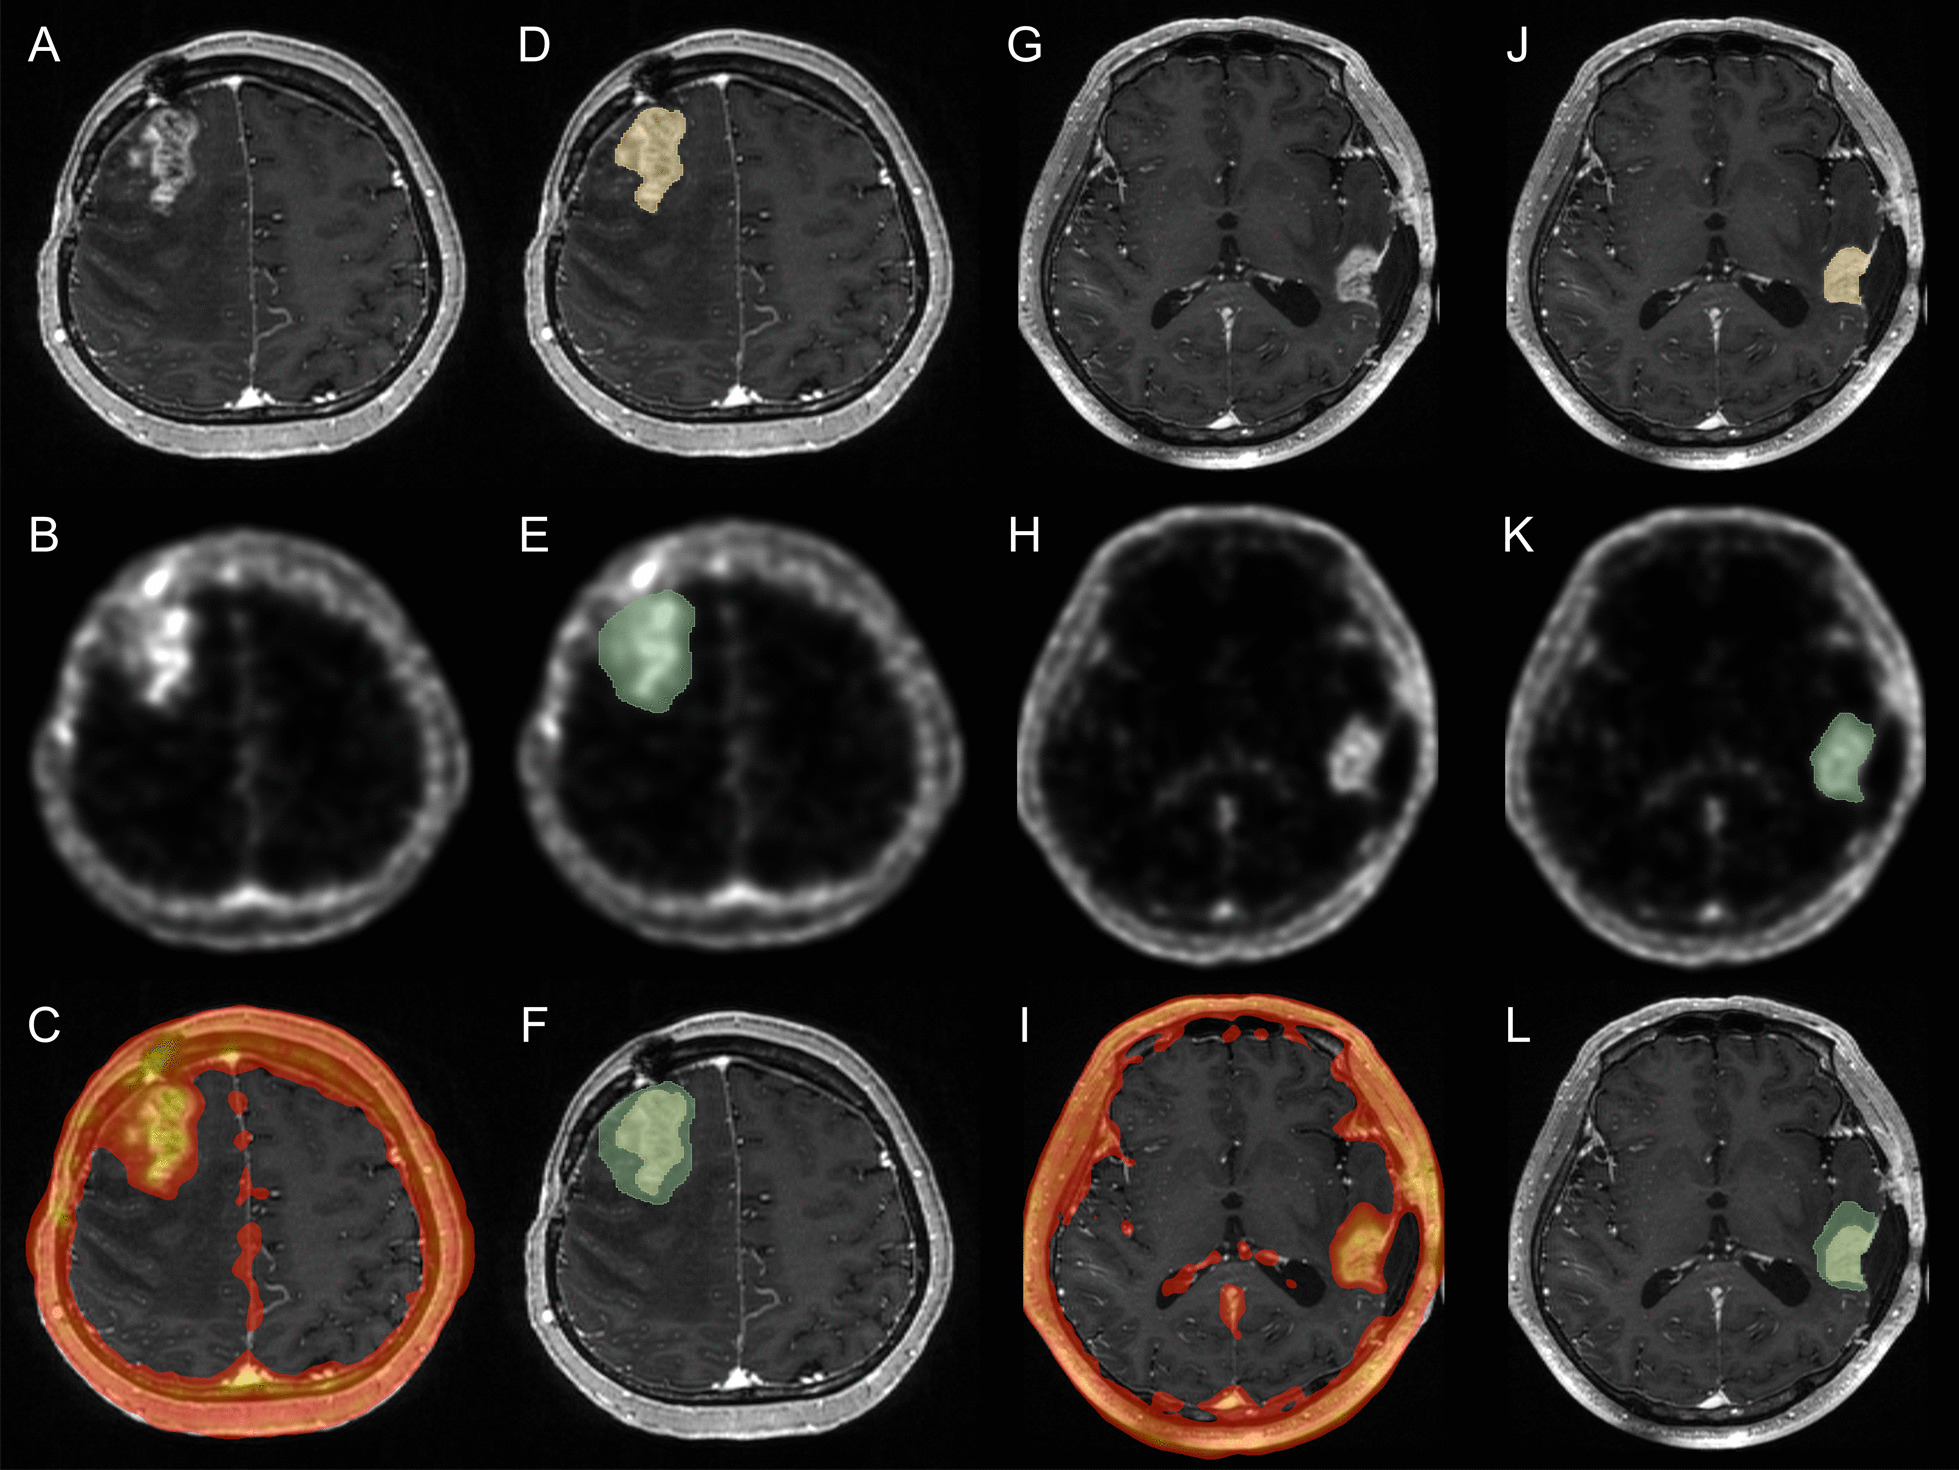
Examples of MRI and FBY PET images in diffuse tumors. A right frontal recurrent WHO grade III oligodendroglioma (IDH mutant, chromosomal 1p/19q codeletion) displayed significant contrast enhancement (A) and FBY activity (B), with increased FBY uptake seen beyond contrast enhancement in the lateral and posterior regions (C). Semiautomated segmentation based on MRI (D) and FBY PET (E) was performed, and nonconcentric expansion of the FBY-based segment compared with the MRI-based segment was noted (F). Similarly, a left temporal recurrent WHO grade IV glioblastoma (IDH-wild type) exhibited contrast enhancement (G) and FBY radioactivity (H), with the increased FBY uptake extended anteriorly and medially (I). The semiautomated segmentations (J, K) also verified the extension of the ROI_FBY_ to the potential invaded regions (with an abnormal T2-weighted signal). This characteristic may result from the infiltrative nature of diffuse gliomas and indicates that FBY can be absorbed in both contrast-enhanced regions and infiltrative noncontrast-enhanced tumors
